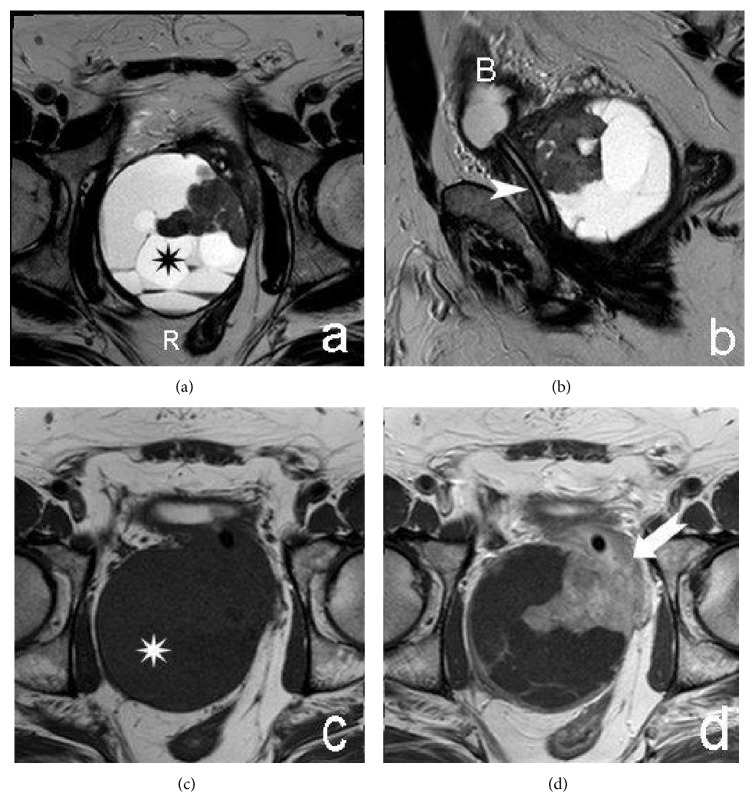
MRI. Axial (a) and sagittal (b) T2-weighted images. Axial precontrast (c) and postcontrast (d) T1-weighted images. The asterisk is on the cystic component of the prostatic lesion; septa are evident. The arrow shows the solid component that improves after Gadolinium injection. Note the presence of a urinary catheter (arrowhead). B = bladder; R = rectum.
